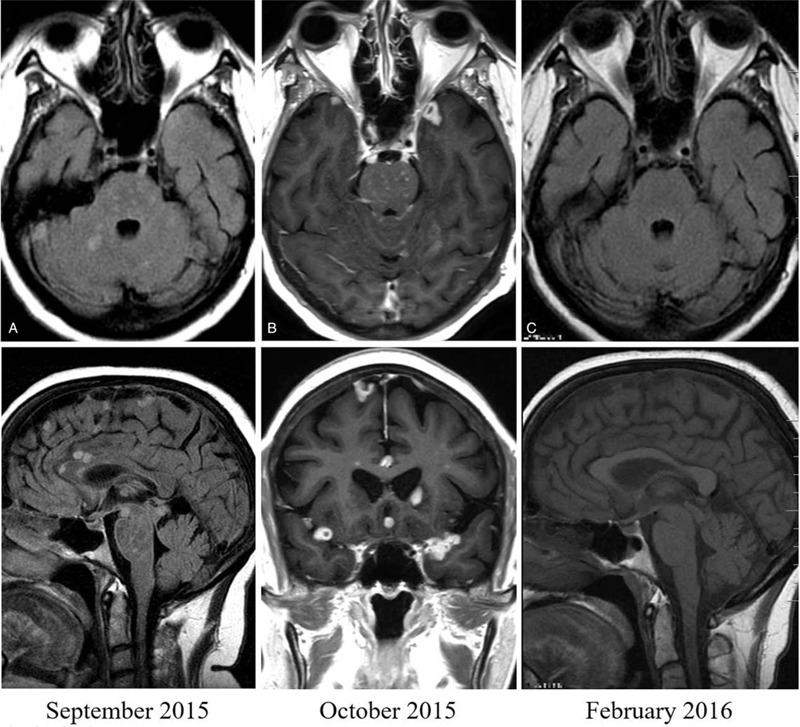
Magnetic resonance imaging of case 1. Axial and sagittal fluid-attenuated inversion recovery images without gadolinium (A) and axial and coronal T1-weighted images with gadolinium (B) performed 5 (September 2015) and 8 weeks (October 2015) respectively after starting tuberculosis treatment, showing multiple supra-and infratentorial lesions, distributed in the brain parenchyma and subarachnoid space. Axial and sagittal scans without gadolinium (C), taken after 6 weeks after receiving a three-dose course of infliximab, showing complete resolution of the tuberculomas.
